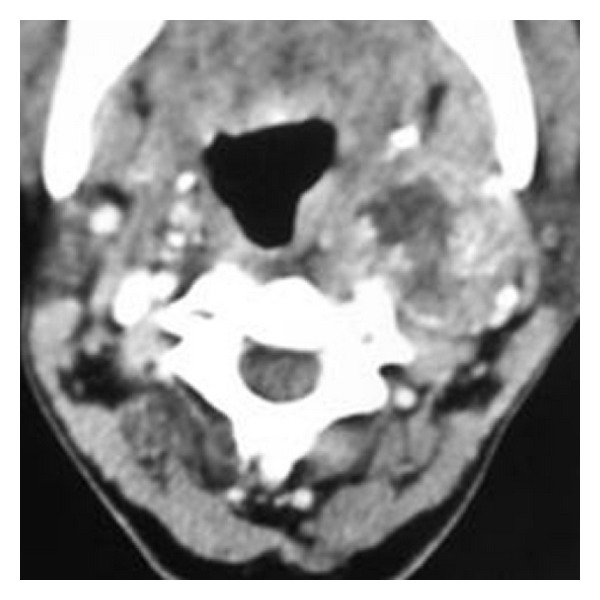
Computed tomography of the neck shows heterogeneously enhancing hypodense mass in left carotid space causing splaying of carotid bifurcation.
